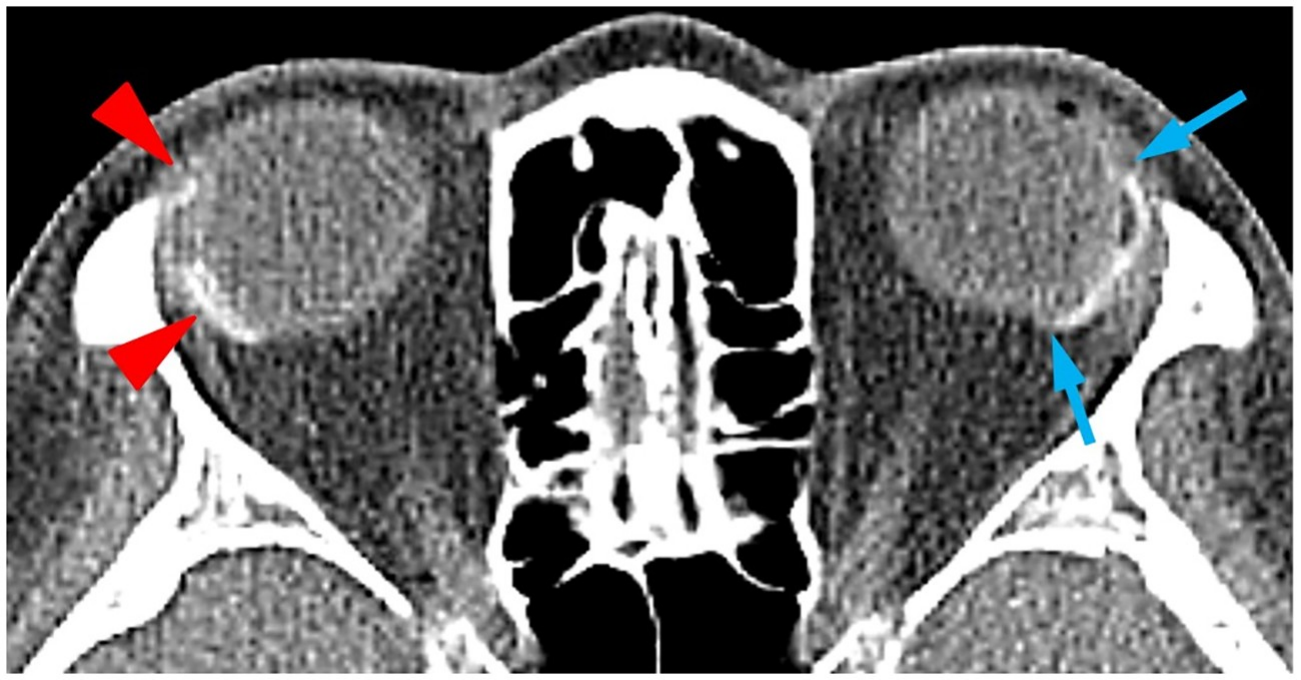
A facial computed tomography image of a patient who underwent Ahmed glaucoma valve (AGV) implantation in both eyes. In our study, one patient underwent two separate implantations in either eye. Initially conventional AGV implantation was performed on the right eye (arrowhead) and free-plate AGV implantation performed on the left eye (arrow) 3 years later. One year after the final surgery, we obtained a facial computed tomography scan to verify implant locations; both plates were safely positioned (well posterior to the equator) without any notable difference in location.
